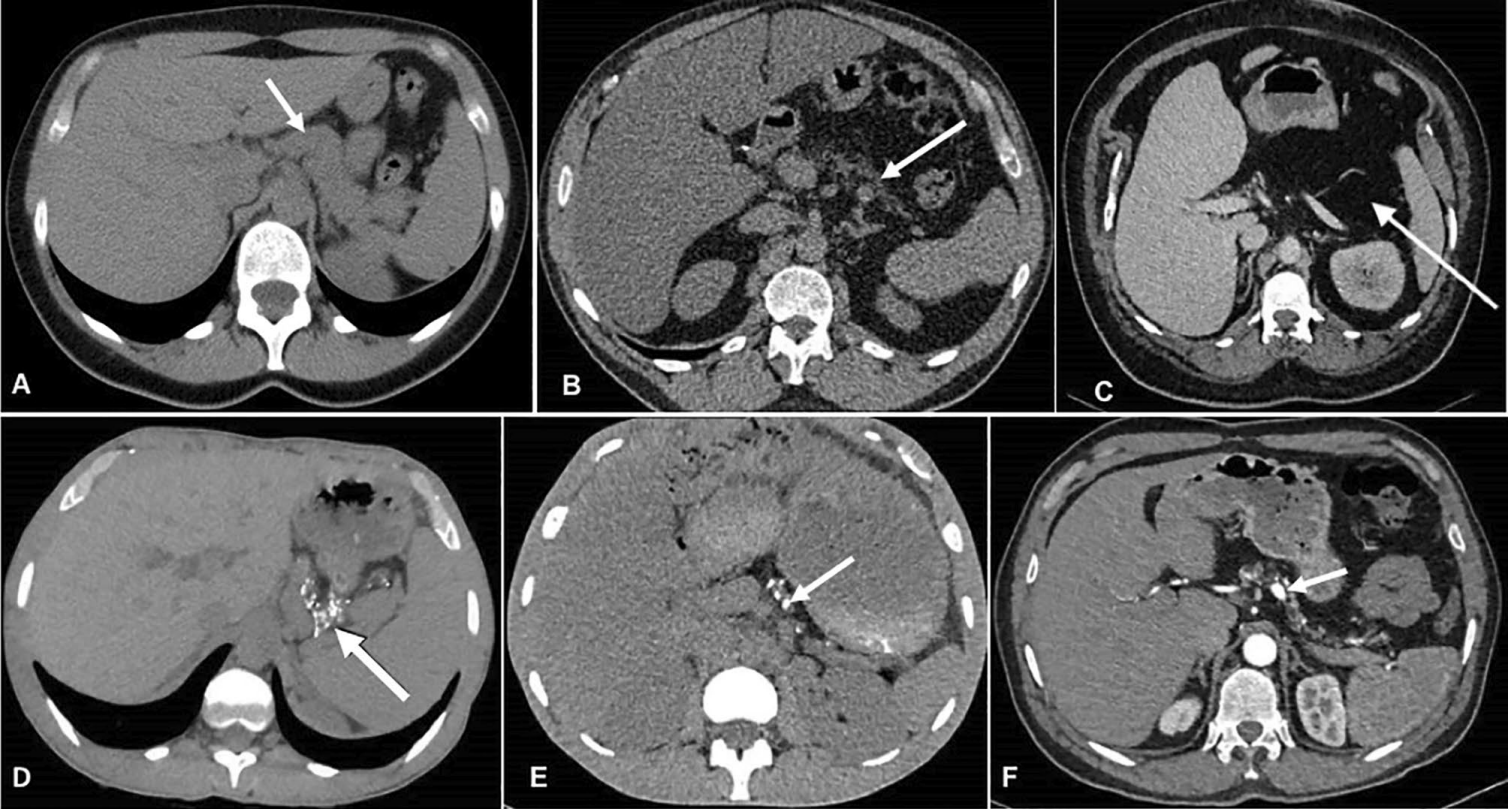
Computed tomography without contrast Top row: Different degrees of pancreas lipomatosis. (a) Normal pancreas (b) Partial lipomatosis of the pancreas. (c) Complete lipomatosis of the pancreas (white arrow shows the visually empty pancreatic area.) Bottom row (d–f): three examples of pancreatic calcifications (arrows). Of note, all images of bottom row show partial lipomatosis of the pancreas.
